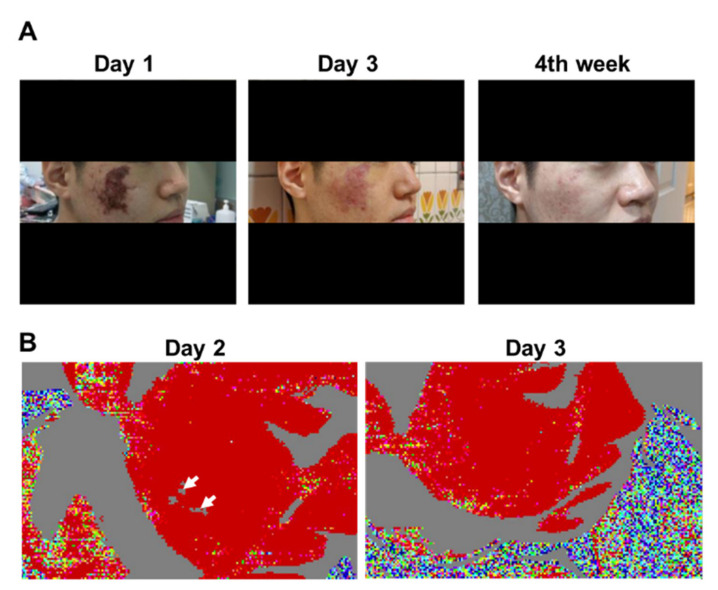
Clinical photographs and laser Doppler imaging of Patient 6. (A) Day 1 of admission; Day 3 of admission; Week 4 of follow-up. Gradual improvement in cutaneous symptoms can be observed. (B) Left panel: on Day 2 of admission, areas of cutaneous ischemia were clearly delineated from those without vascular occlusion. Right panel: on Day 3 of admission, facial blood circulation was restored.
